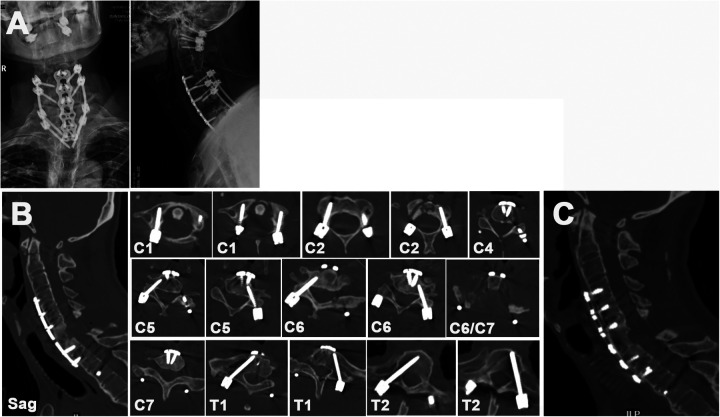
(A) postoperative cervical x-ray image. (B) Postoperative cervical CT image 2 days after surgery. (C) Postoperative cervical sagittal CT image 9 months after surgery showing healing of the odontoid fracture and bone fusion after C6 iliac bone transplantation.
